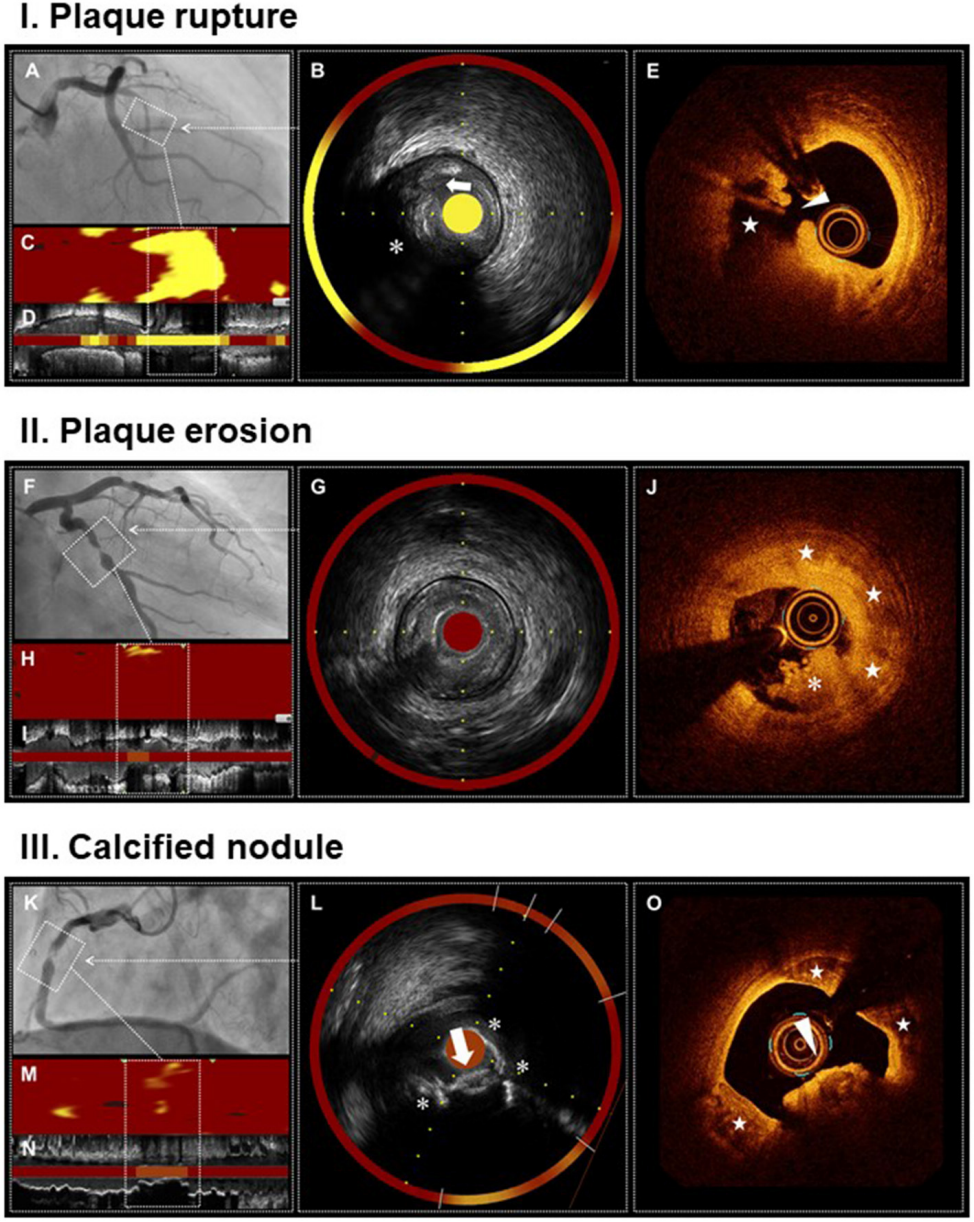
Multimodality imaging of plaque rupture, plaque erosion, and calcified nodule. Plaque rupture (I) Angiogram shows occlusion of proximal LAD, the culprit lesion of STEMI (A). IVUS demonstrates ulceration (arrow) with a recess on the surface of attenuated plaque (asterisk) (B,D). NIRS identifies a large lipid content (maxLCBI4mm = 920) (B–D). OCT reveals plaque rupture characterized by a fibrous cap disruption (arrowhead) and a cavity (star) formation inside the plaque (E). Plaque erosion (II) Angiogram shows severe stenosis in mid LCX, the culprit lesion of STEMI (F). IVUS demonstrates the absence of plaque ulceration (G,I). NIRS identifies a small lipid content (maxLCBI4mm = 129) (G–I). OCT reveals plaque erosion characterized by the presence of attached thrombus (asterisk) overlying an intact fibrotic plaque (stars) (J). Calcified nodule (II) Angiogram shows severe stenosis and intraluminal filling defect in mid RCA, the culprit lesion of non-STEMI (K). IVUS demonstrates a convex calcium with irregular surface (arrow) and large superficial calcium (asterisks) (L,N). NIRS identifies a moderate lipid content (maxLCBI4mm = 208) (L–N). OCT reveals calcified nodule characterized by a protruding calcium with thrombi (arrowhead) and large superficial calcium (stars) (O). IVUS, intravascular ultrasound; LAD, left anterior descending artery; LCBI, lipid core burden index; LCX, left circumflex artery; NIRS, near-infrared spectroscopy; OCT, optical coherence tomography; RCA, right coronary artery; STEMI, ST-segment elevation myocardial infarction.
